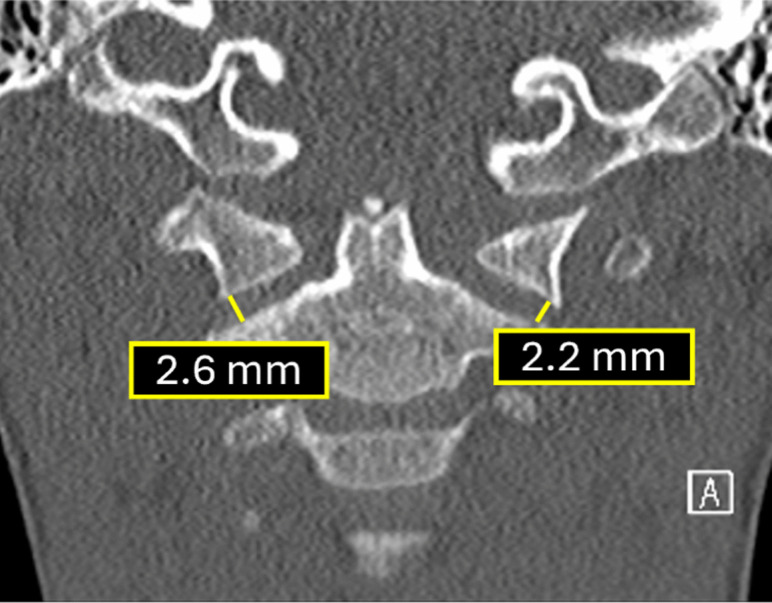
Coronal CT in a 4-year-old child demonstrates normal atlanto-axial interval
